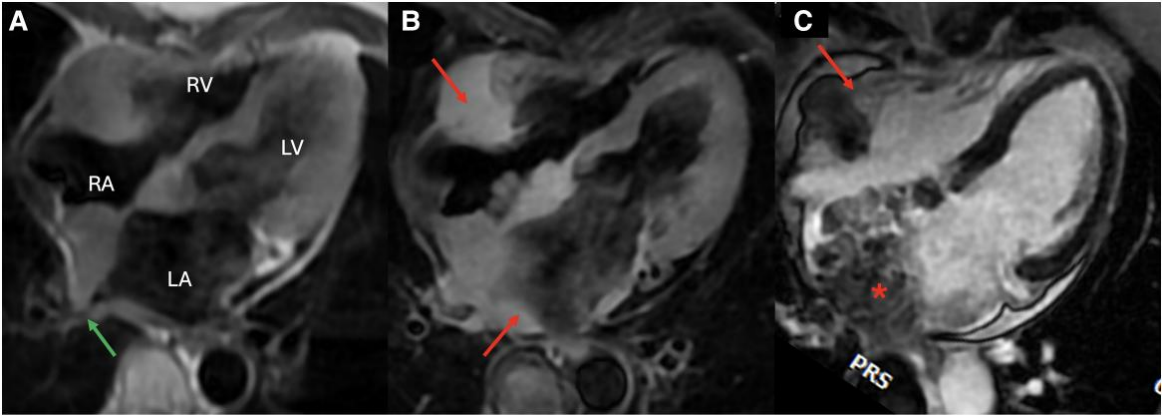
Cardiac magnetic resonance depicts a large cardiac mass measuring 11 × 6.3 cm, with irregular borders and extensive infiltration of both atria and adjacent structures (arrows in B and C). Invasion of the right pulmonary vein ostium is noted, with associated narrowing of the right inferior pulmonary vein (arrow in A). On tissue characterization, the mass exhibits features suggestive of malignancy: (*A*) T1-weighted imaging: the mass is isointense and shows no signal suppression on fat-saturated sequences. (*B*) T2-weighted imaging: the mass is hyperintense, consistent with increased water content and oedema. (*C*) Late gadolinium-enhanced imaging: heterogeneous contrast uptake is observed, with extensive infiltration of the left atrium (asterisk).
